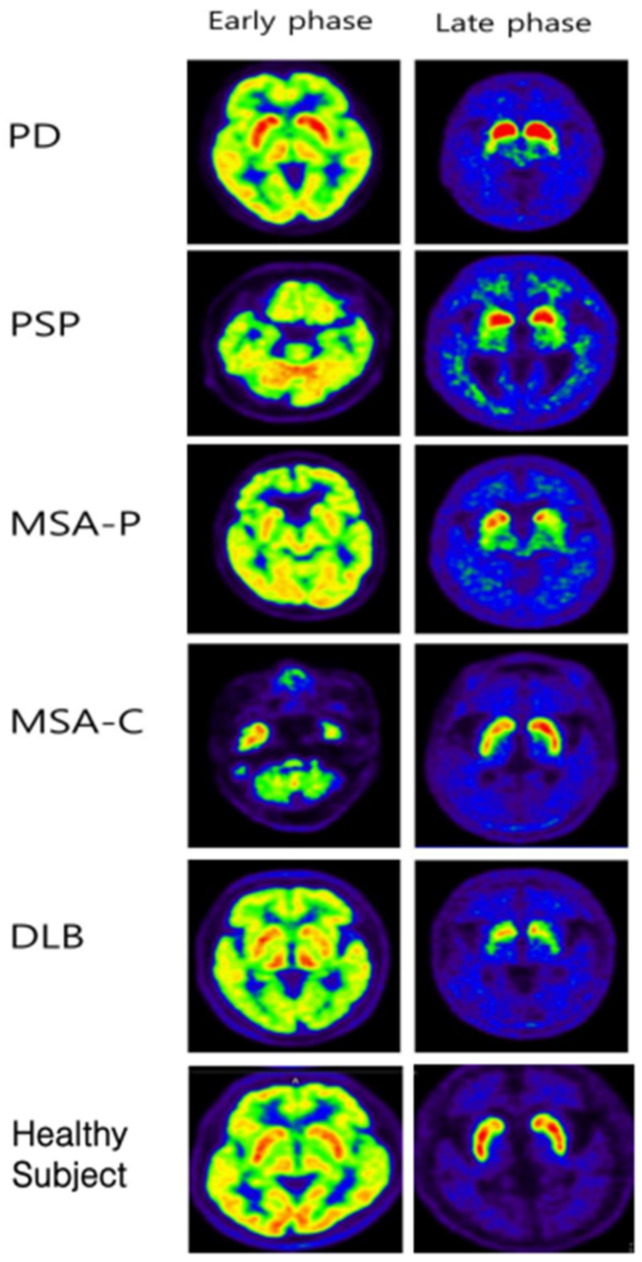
Representative images of early and late phases ^18^F-FP-CIT PET/CT from PD, PSP, MSA-P, MSA-C, and DLB patients. In the late phase, even in APDs such as DLB, a rostocaudal gradient was observed, and in MSA-C patients, the asymmetric index was increased. In other words, it can be said that it is difficult to differentiate between PD and APS using only the late phase image.
